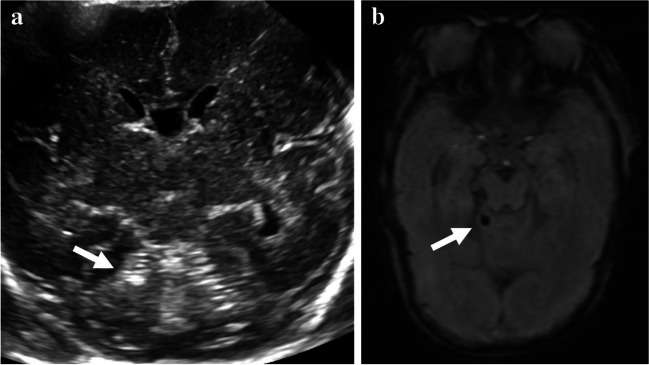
Initially missed but retrospectively detected type 1 cerebellar hemorrhage in a preterm boy (27 + 5 weeks, 1,080 g). a Oblique coronal anterior fontanelle view of cranial ultrasound image shows an echogenic punctate hemorrhage (*arrow*) in the right upper peripheral cerebellum, which was initially overlooked in the report, but was later discovered upon retrospective image review. b Axial susceptibility-weighted magnetic resonance image shows a tiny hemorrhagic focus (*arrow*) in the right upper peripheral cerebellar hemisphere
